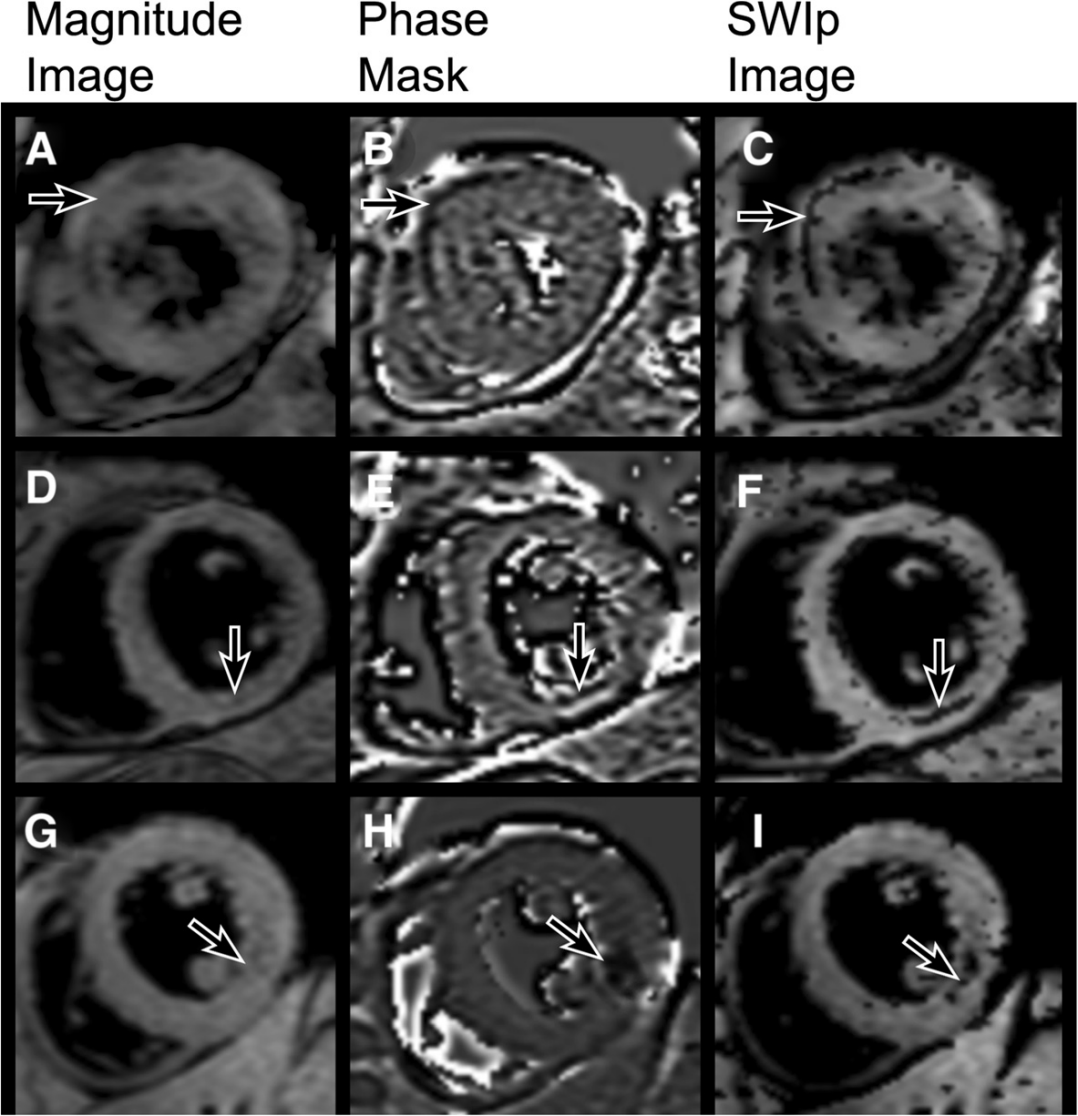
Contrast generation by SW MRI in three different patients. Top row: Anterior AMI with IMH (arrowed) is shown in magnitude image without susceptibility weighting (A). A phase mask is generated using the SWIp method (B) and applied to the magnitude image to generate SW images with additional contrast for IMH (C). A similar process is shown for IMH in inferior (D-F) and inferolateral (G-I) territories.
